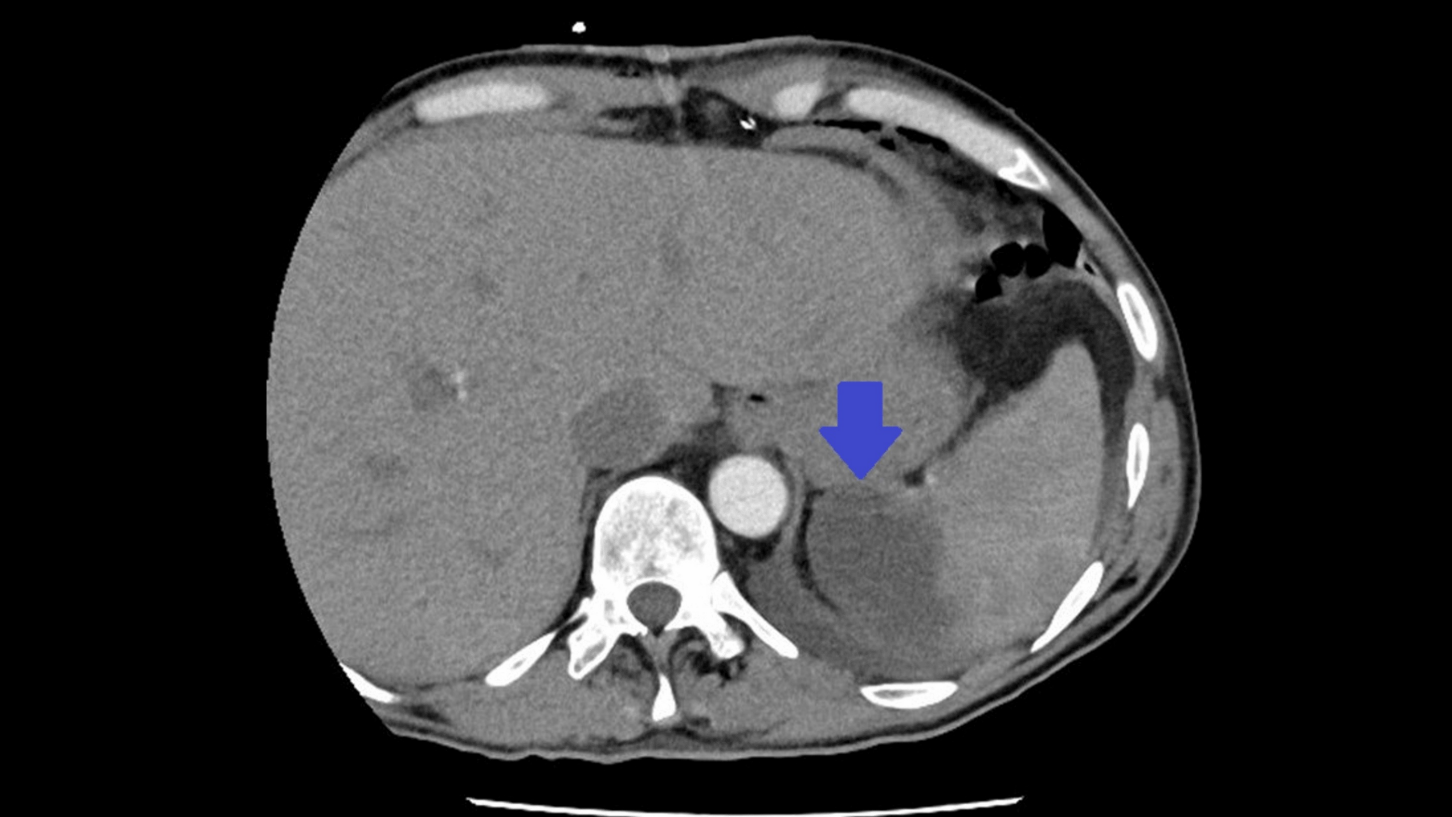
Abdominopelvic CT scan showing splenomegaly and splenic infarction (blue arrow).
